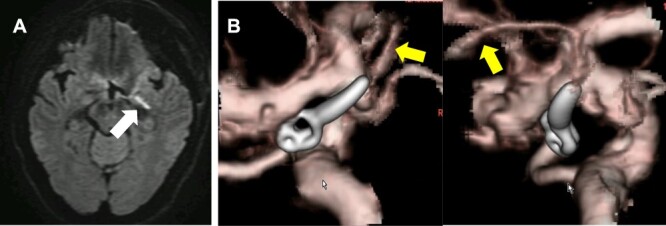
DWI-MRI revealed a new ischemic brain lesion at the uncus of the left AchA territory 1 day after surgical clipping (A). CTA demonstrated complete aneurysm obliteration and a healthy patency of the AchA main trunk (B, yellow arrow).
